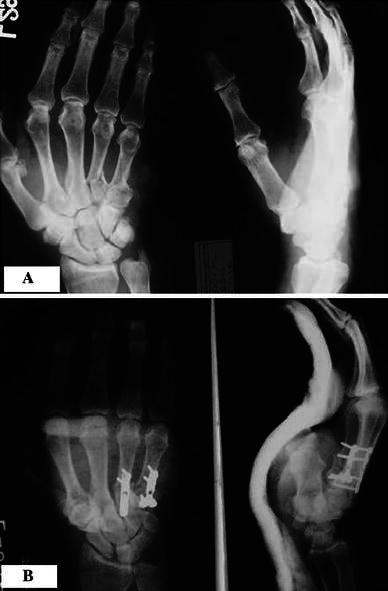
Case 1: a preoperative and b postoperative X-rays
